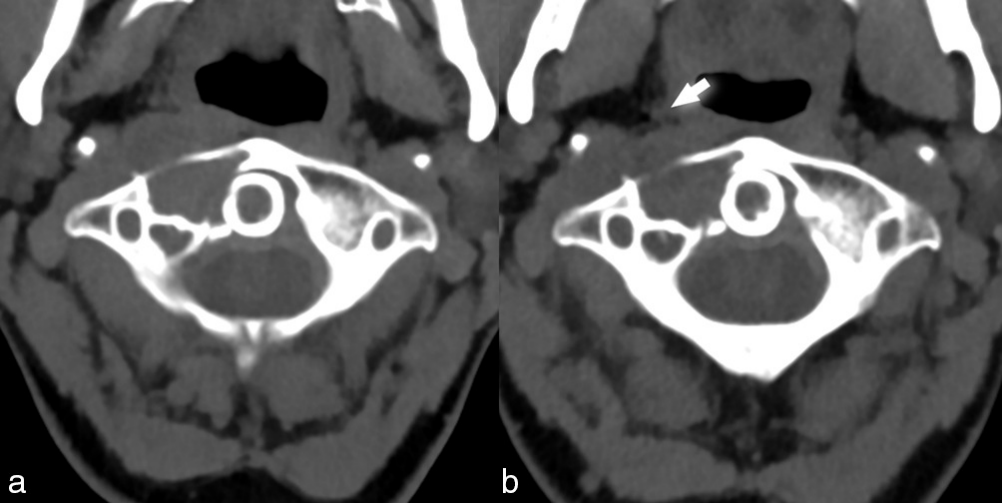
Treatment changes in a 49-year-old female with pigmented villonodular synovitis of the cervical spine. Non-contrast-enhanced CT scan performed pretreatment (a) and 3 months following the initiation of imatinib mesylate therapy (b) reveals subtle reduction in the lesion size, best appreciated in the prevertebral component of the perivertebral space. The reduction in soft-tissue density mass with a more normal contour of the right parapharygeal space (white solid arrow) on post-treatment imaging is noteworthy.
